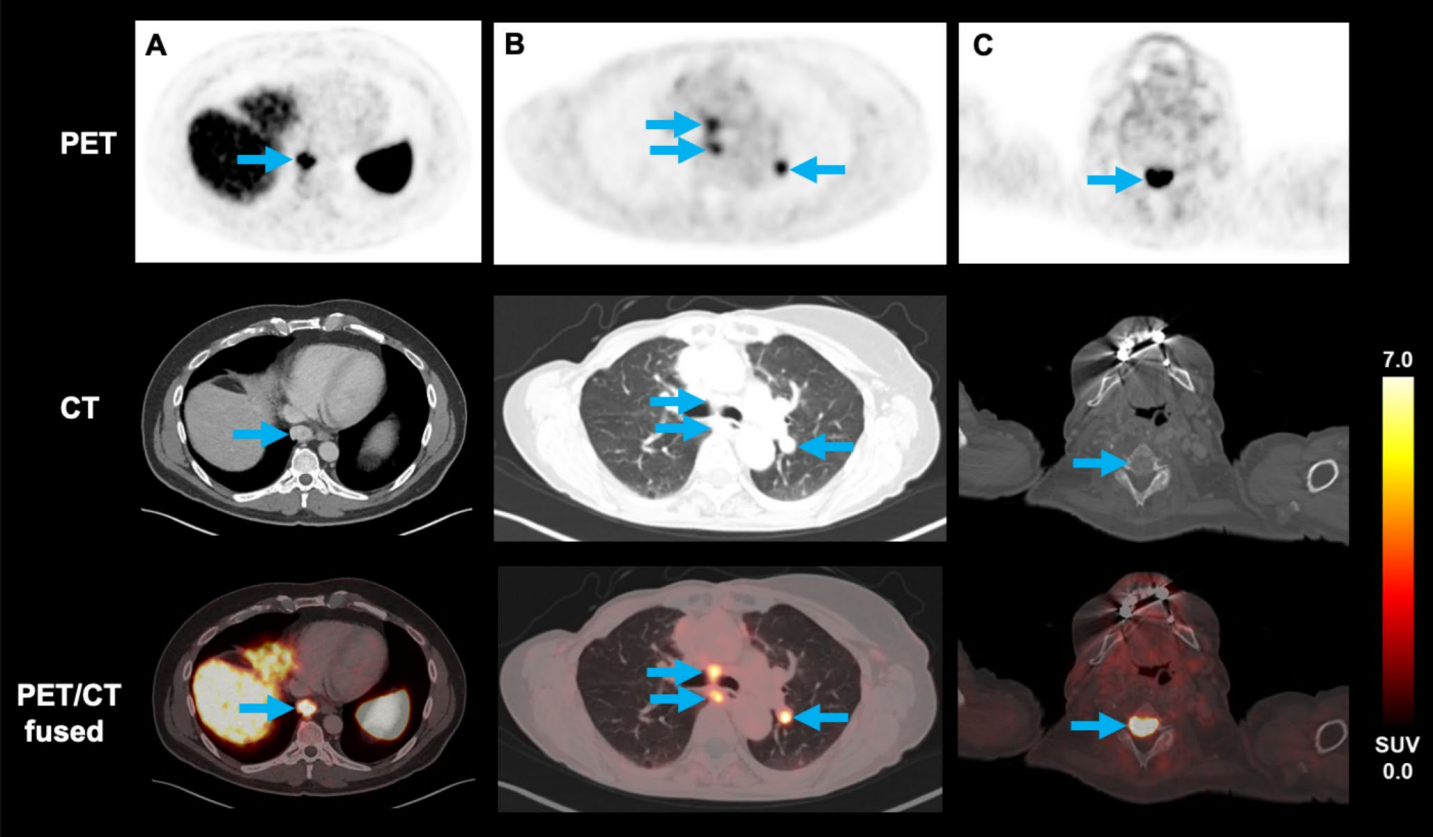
PET/CT images using the novel radiotracer [^18^F]SiTATE in patients with a mediastinal metastasis of MTC (A), with nodal and pulmonary metastases of FTC (B) and an osseous metastasis of FTC (C)
